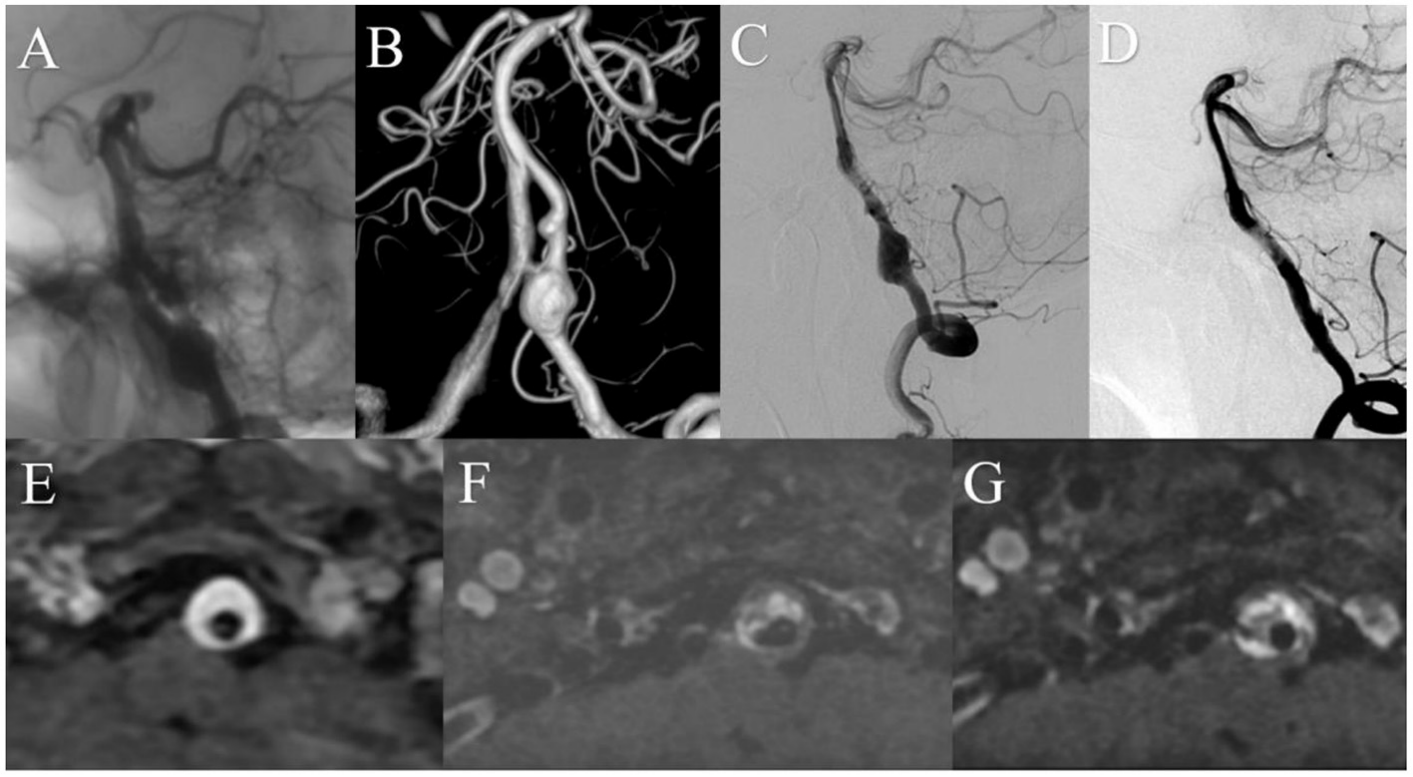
Preoperative anteroposterior (A) and three-dimensional reconstruction (B) digital subtraction angiography (DSA) showed a left vertebral artery aneurysm. (C) DSA immediately after treatment showed that contrast stasis within the aneurysm. (D) One year after treatment, DSA showed satisfactory arterial reconstruction and complete aneurysm obliteration. Magnetic resonance imaging demonstrated stability of the intramural hematoma over time [(E) before treatment; (F) 1 year after; (G) 3 years after].
